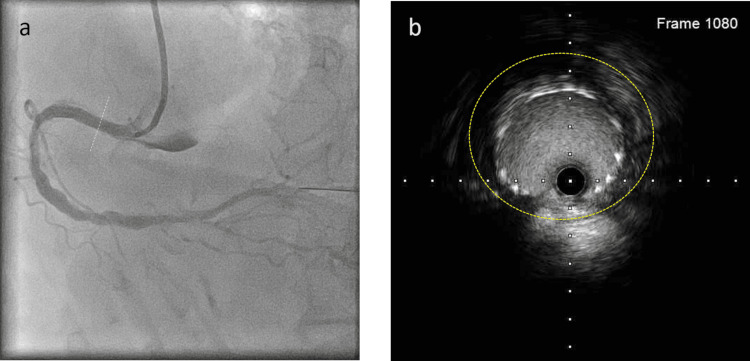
Final coronary angiography and cross-sectional intravascular ultrasound image. a: The white dotted line in the right coronary angiography indicates the location of the corresponding cross-sectional intravascular ultrasound image. b: Cross-sectional intravascular ultrasound image showing the stent was deployed roundly; however, a hematoma remained entirely around the stent (yellow dots).
